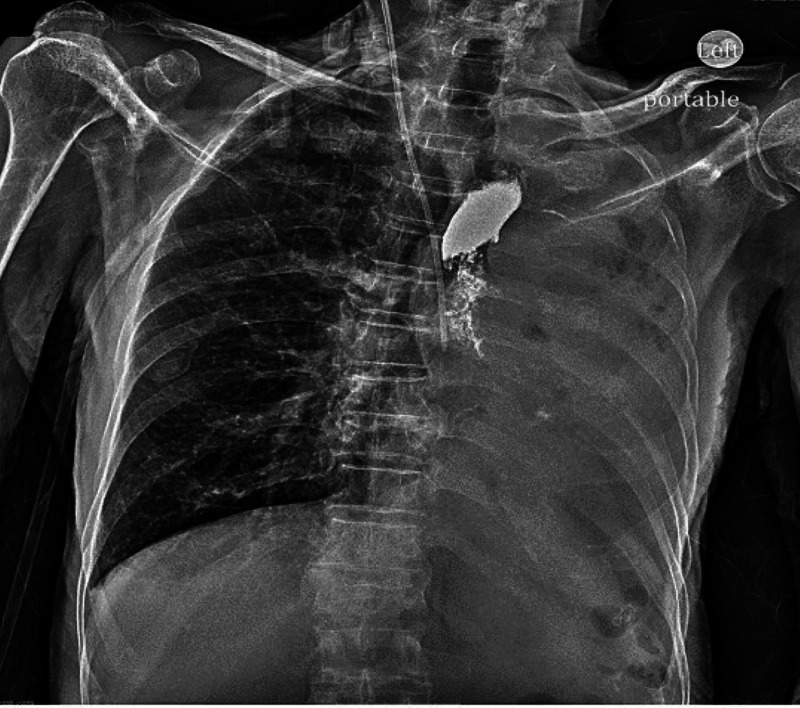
Chest X-ray revealed interval development of complete opacification of left hemithorax and retained barium in the left upper chest.
